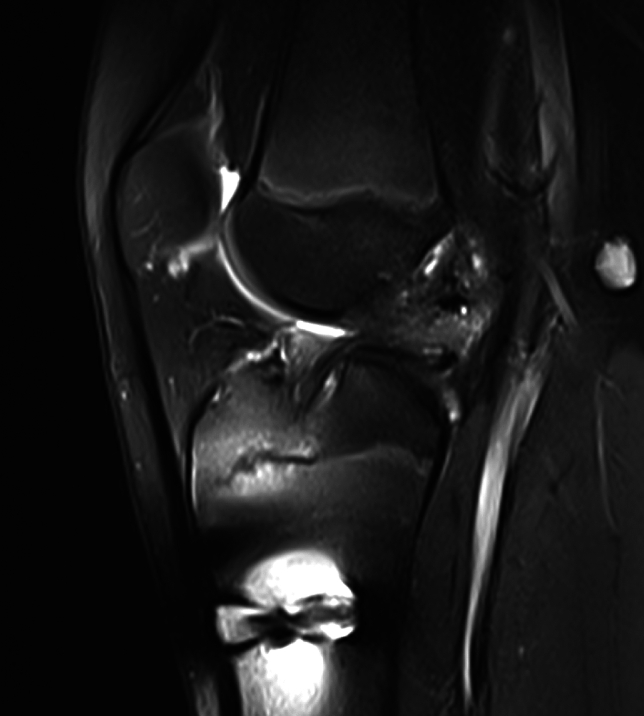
16-year-old male patient at follow-up MRI 5 months after QT autograft ACLR showing complete graft resorptionand missing graft signal 5
